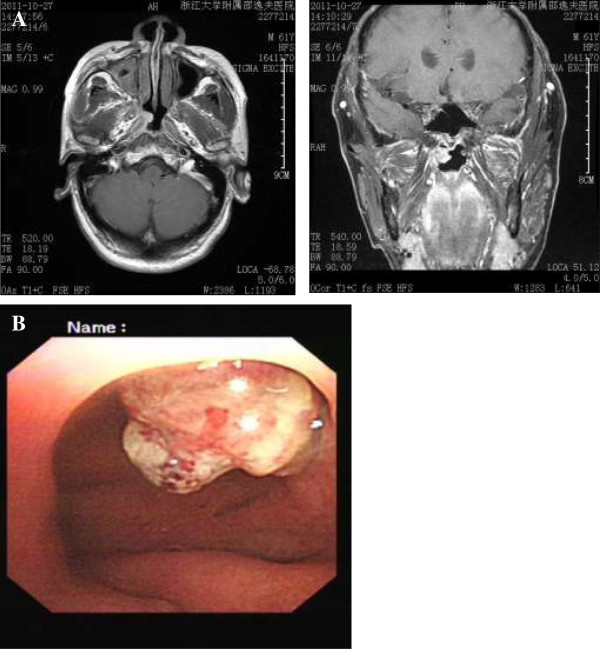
Scans show the mass in the torus tubarius of the nasopharynx. (A) Magnetic resonance imaging scan. (B) Bronchoscopy.
